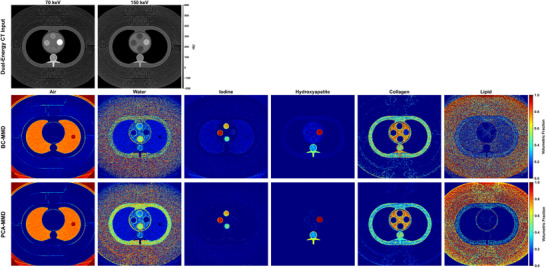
Visualization of material fraction maps for six basis materials (air, water, iodine, hydroxyapatite, collagen, and lipid) obtained from dual‐energy PCCT images reconstructed at 70 and 150 keV. Top row: input CT images (WW/WL = 400/50 HU). Middle row: BC‐MMD decomposition results. Bottom row: PCA‐MMD decomposition results.
